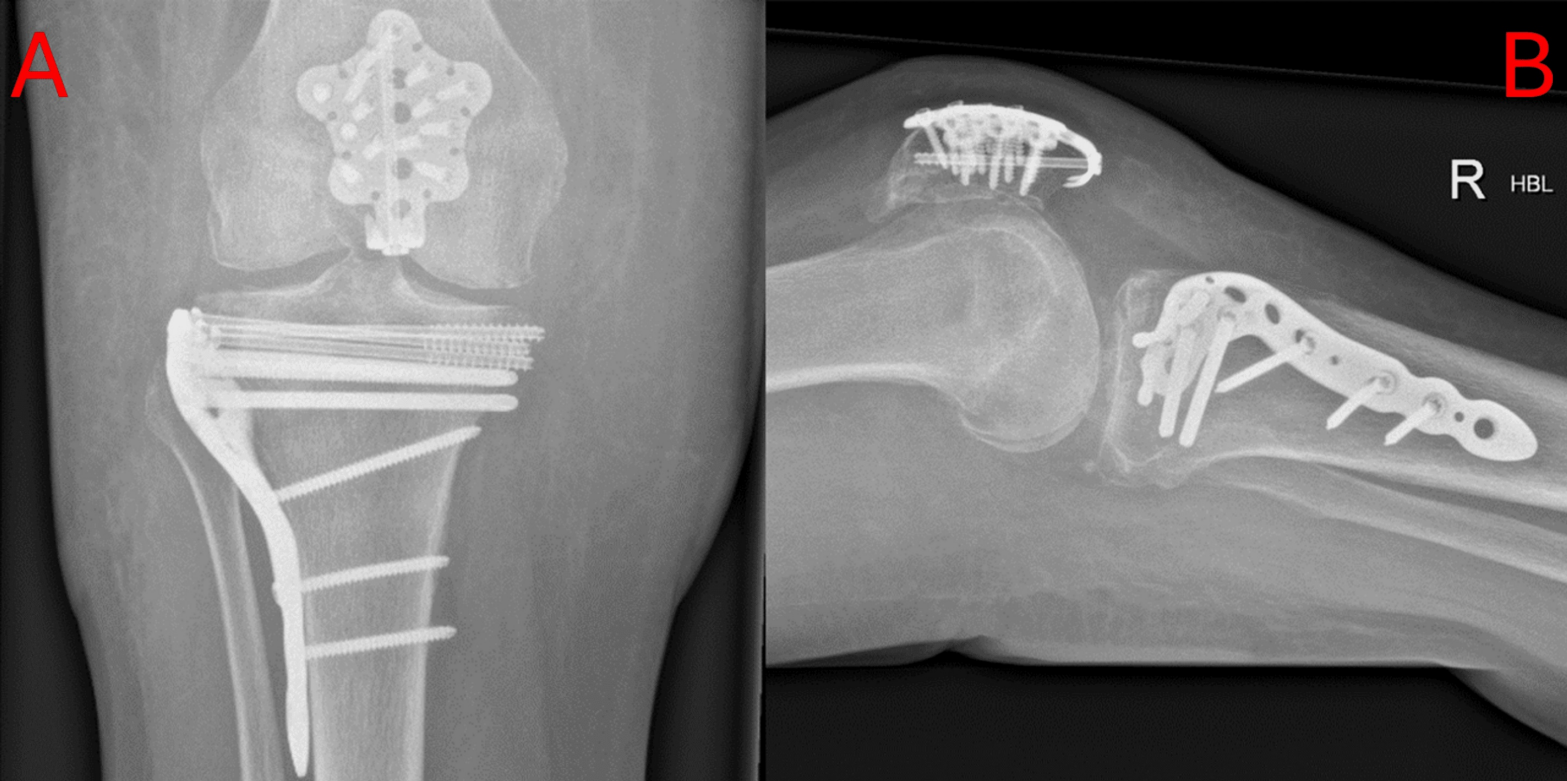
Anterior to posterior (a) and lateral (b) X-rays of right knee three months postoperatively. A) Plain anterior to posterior radiograph of the patient's right knee. This image shows adequate fixation of both the patella and the tibial plateau fracture at three months after the operation; B) Plain lateral radiograph of the patient's right knee. This image shows adequate fixation of both the patella and the tibial plateau fracture at three months after the operation.
